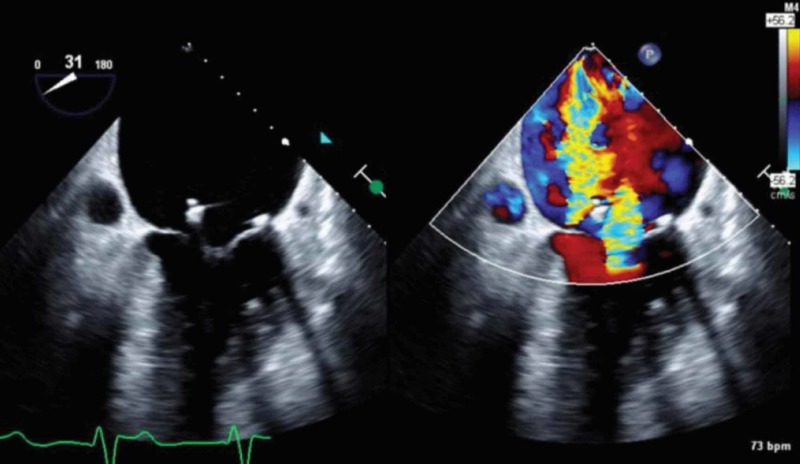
Severe mitral regurgitation noted on 2D TEE image (Left) of the mitral valve at 30-degree mid-esophageal view. Color Doppler with regurgitant jet (Right). TEE, transesophageal echocardiogram
